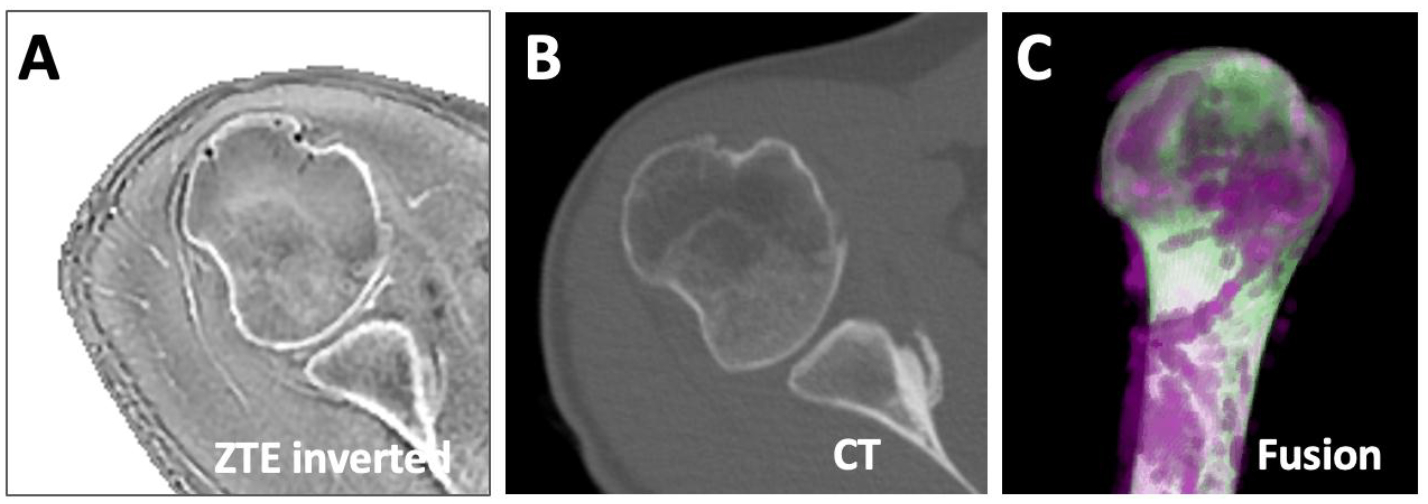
Comparison of MRI vs. CT segmentation. ZTE MRI (A) and CT (B) data of the same subject were registered and segmented (using U-Net for MRI, manually for CT). The segmented images were fused (C), showing the overlapping regions as white, and the non-overlapping regions in magenta for MRI and green for CT.
